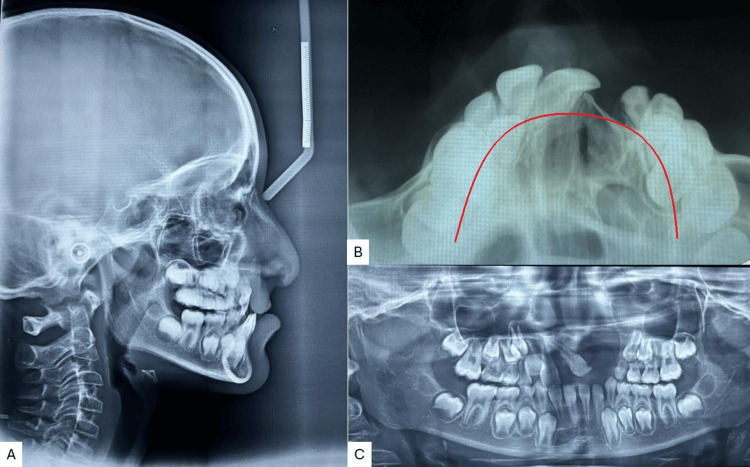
Post-expansion radiographs A) Lateral cephalogram; B) maxillary occlusal radiograph; C) orthopantomogram (OPG)
